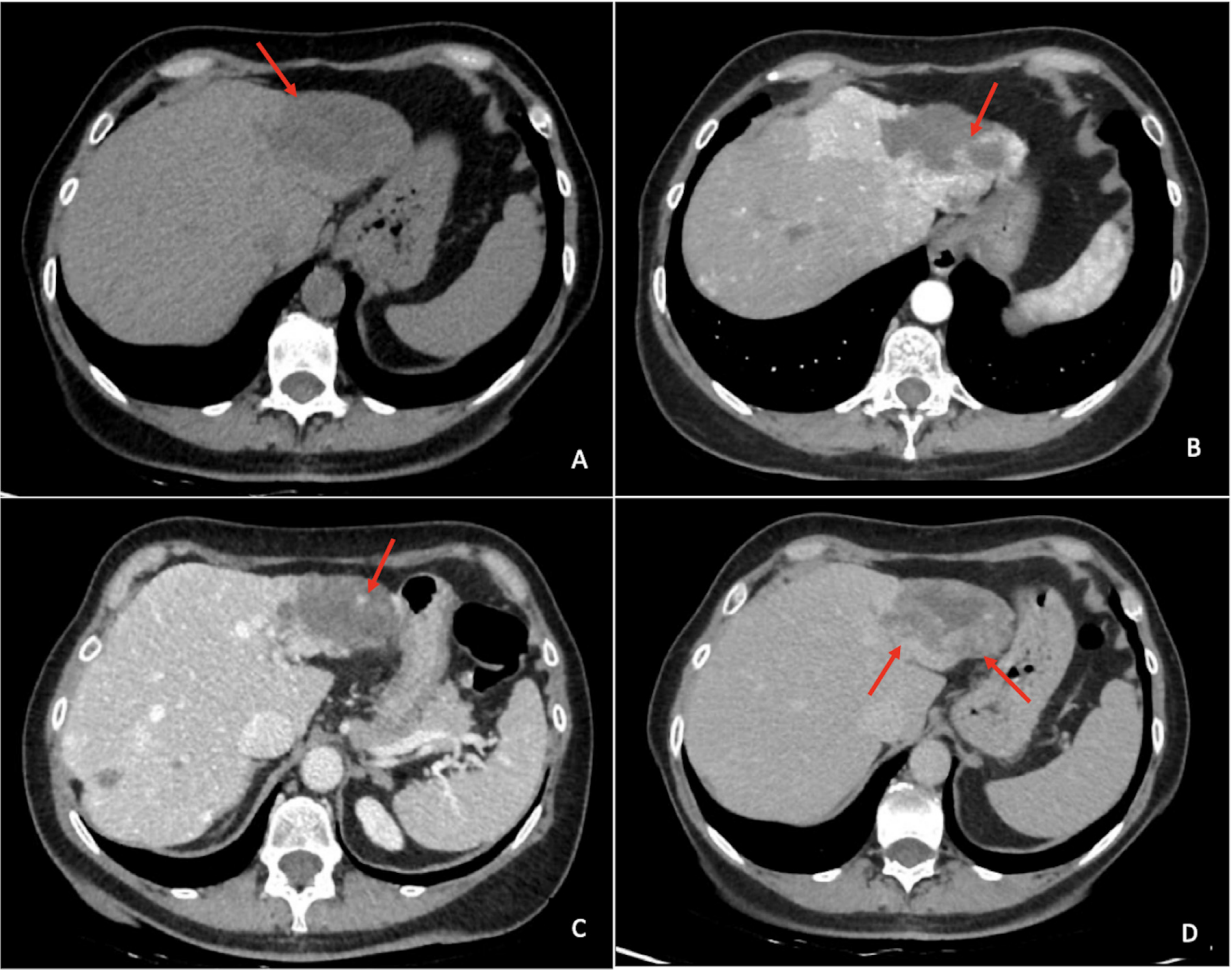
Computed tomography images. An area with polycyclic margins at the II-III hepatic segment is observed, accompanied by evidence of an 8 cm formation in the context of inhomogeneous density in basal conditions (A). This is further characterised by the presence of central vascular pooling evident in the arterial (B) and portal (C) postcontrastographic phases. Subsequently, progressive, irregular and incomplete enhancement of the peripheral portions is observed in the late phase at 7 minutes, with a centrifugal enhancement pattern (D).
